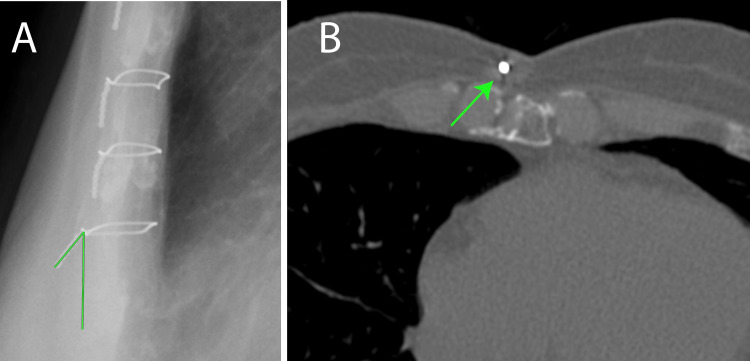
Chest X-p after 14 years (A) Lateral chest X-p demonstrates the sixth wire standing up. The angle between the vertical line is 39.0° (green line). (B) Computed tomography displays no evidence of wire penetration through the skin (green arrow). Chest X-p: chest X-ray photograph
